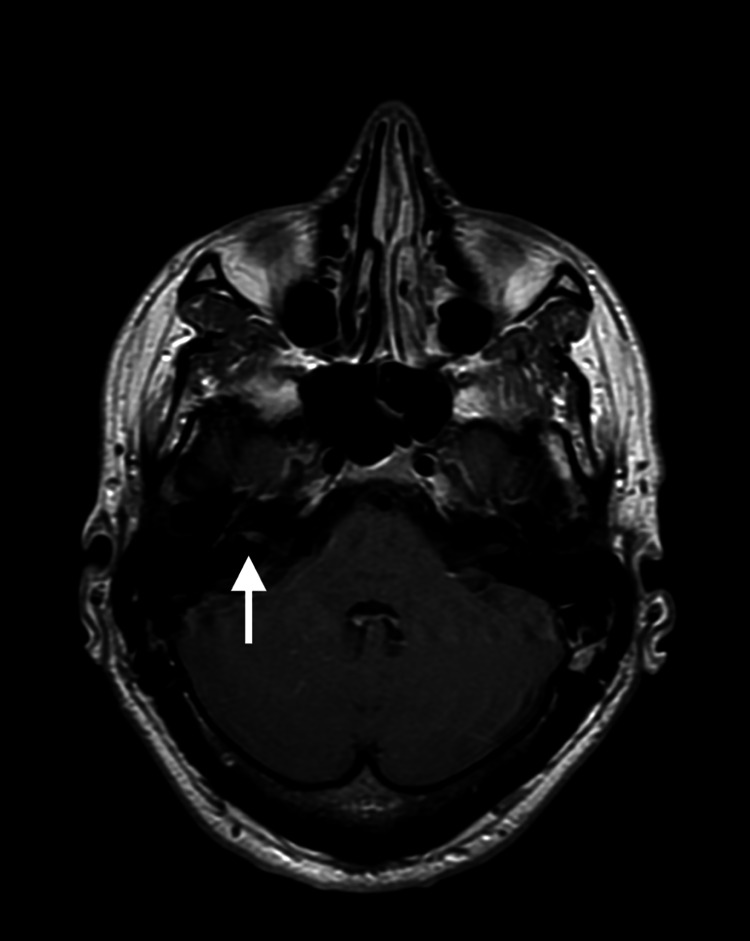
Magnetic resonance imaging (MRI) of the facial nerve Axial T1-weighted postcontrast MRI scan demonstrating enhancement at the right internal acoustic canal (IAC) fundus.
